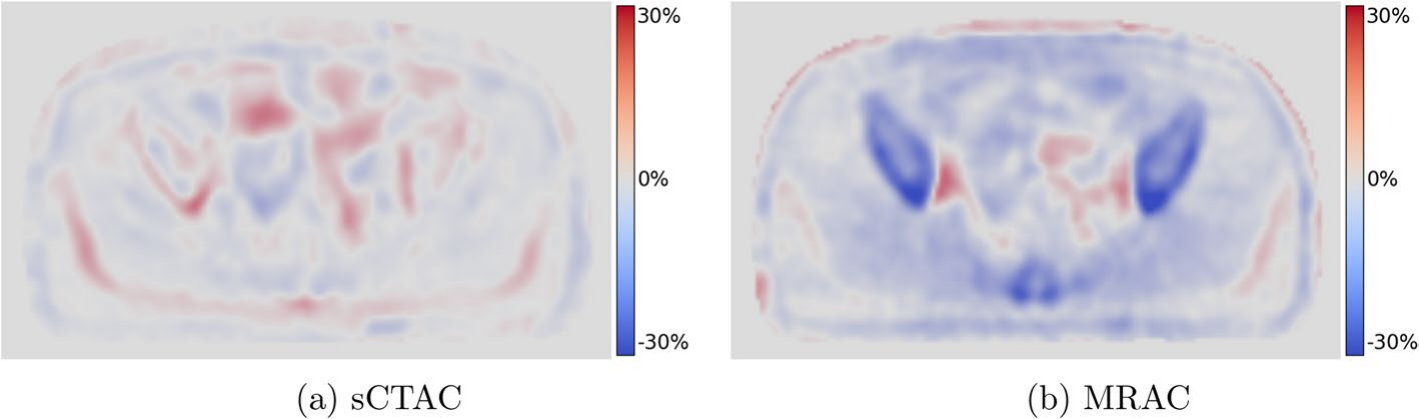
Example SUV difference maps to CTAC PET images for the sCTAC PET image (a) and MRAC PET image (b) for the same patient and slice as shown in Fig. 2
